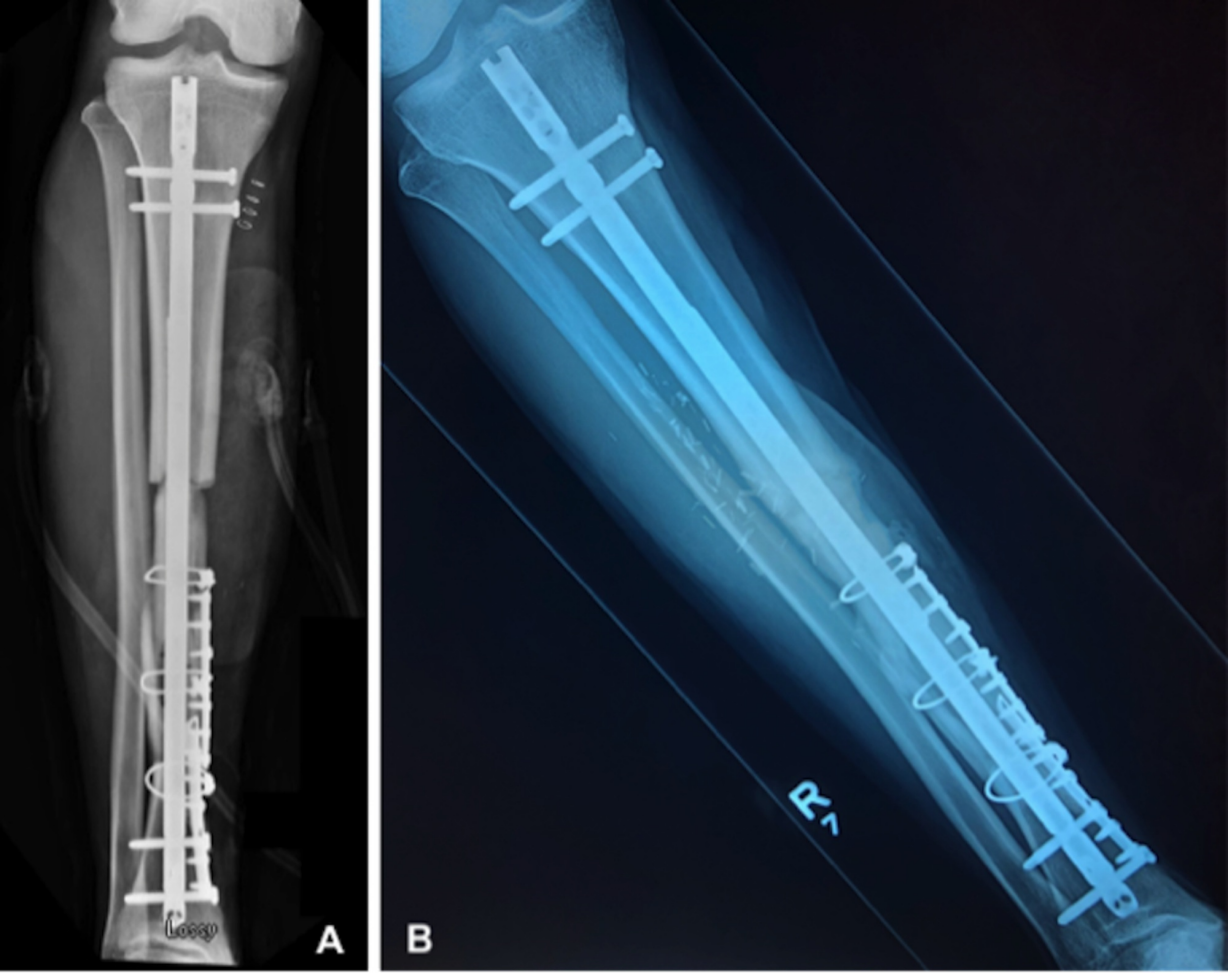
Postoperative radiographs (A) Immediate postoperative anteroposterior radiograph, (B) anteroposterior radiograph at one year follow-up.
